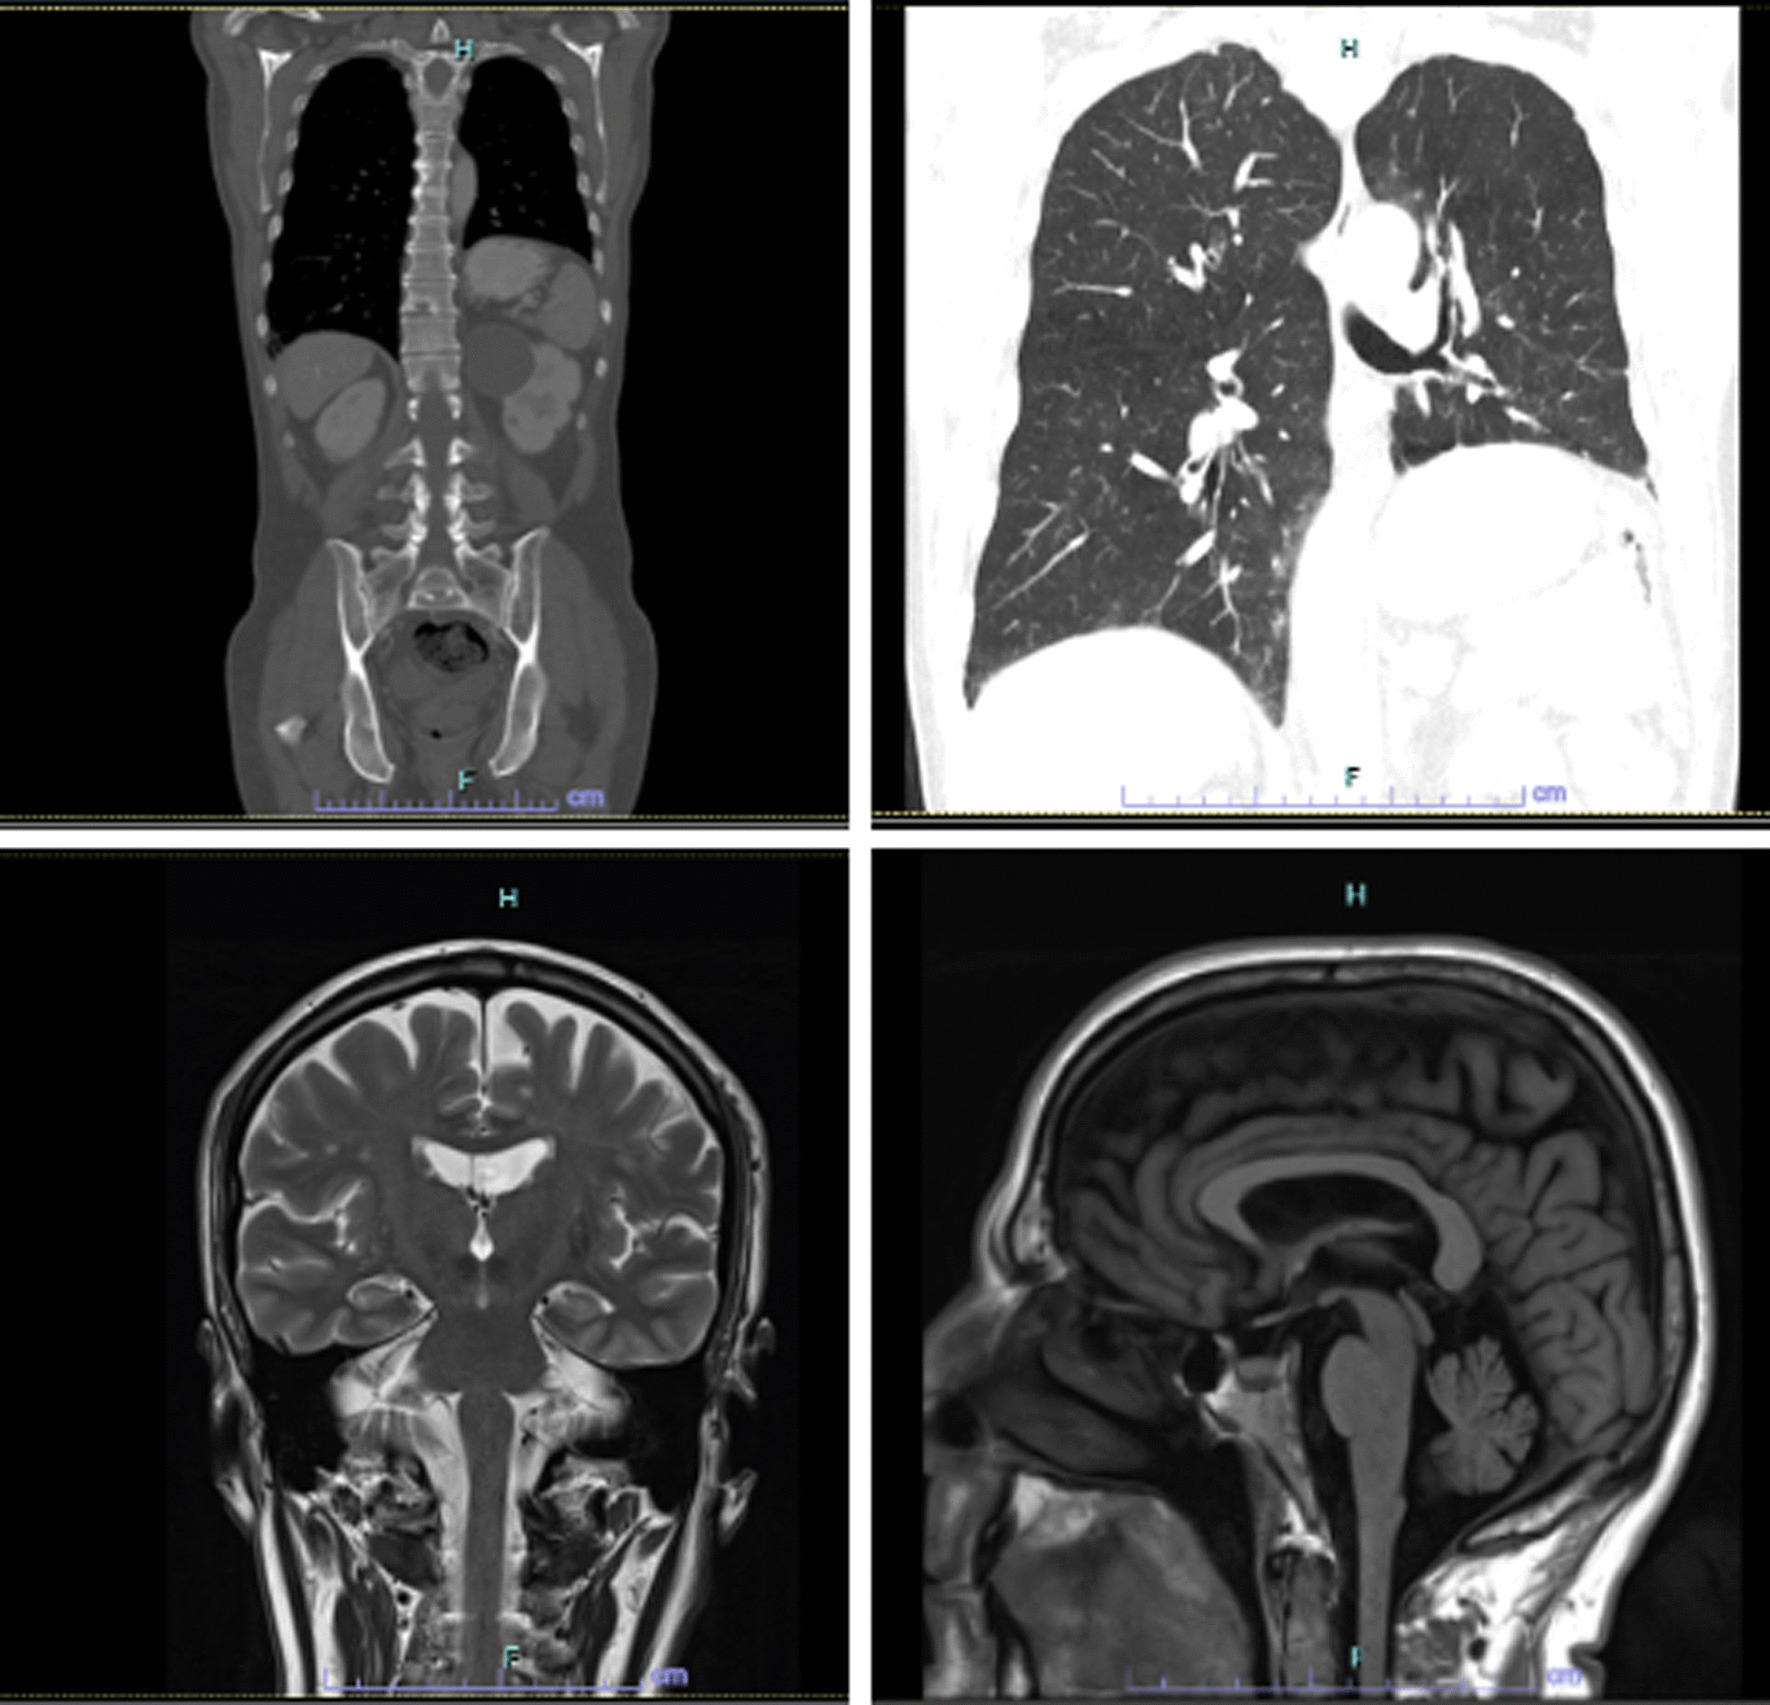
Assessment of response by imaging, Computerized tomography and Magnetic resonance imaging, demonstrating stable disease according to RECIST v1.1 criteria—January 2023
